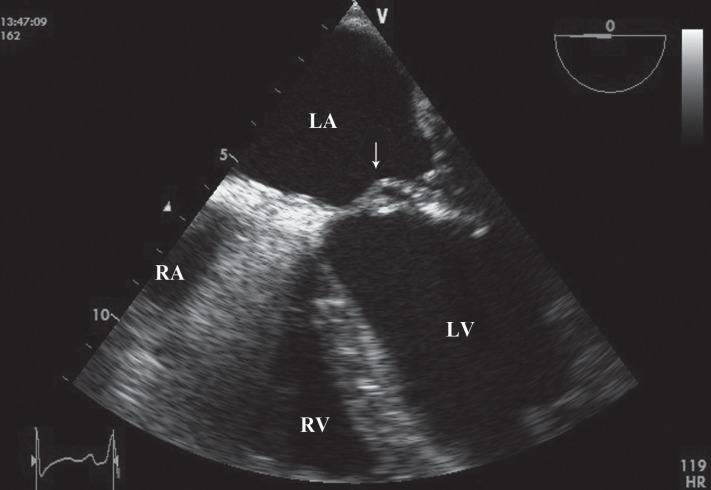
Pseudoaneurysm of the anterior mitral leaflet on transesophageal echocardiography (arrow)
